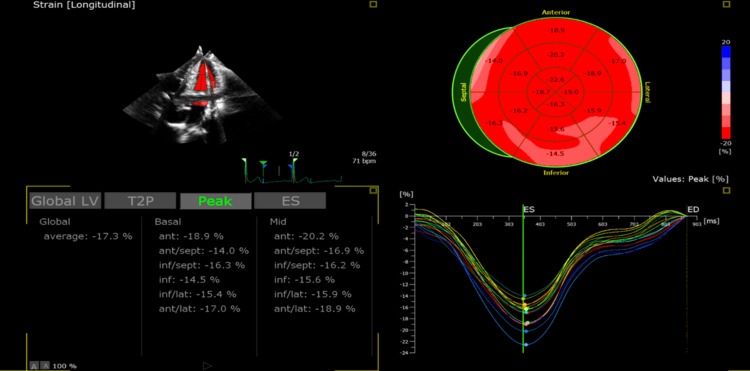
Transthoracic echo echocardiography showed a reduced GLS of 17% with variable regionality. GLS; global longitudinal strain.
